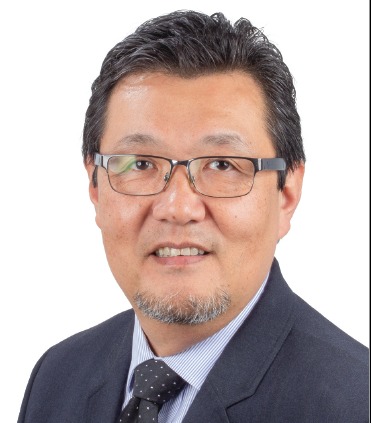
Those who do not know Lincoln Issamu Nojima may have the impression that he is a sedate professor of Orthodontics. However, this great professional has struggled and endured a difficult journey to become the orthodontist that he is today. In the beginning of his career, as he had no dependable advisor, he attended several short-duration courses. He took his first course on typodont in 1989. However, unsatisfied with his learning up to that time, he sought to improve his education by taking other courses to achieve the goal of being a good orthodontist. He even took a course at the Tweed Foundation in 1994. Until one day, his friend Geraldo Queiroz suggested that he should take the Orthodontics course at the Federal University of Rio de Janeiro (UFRJ), which he did. He went on to take a Master’s Degree (1995-1996) and a Doctorate (1999-2004) and started working as a professor in the Department of Orthodontics of UFRJ in 1997. In his search for more knowledge, he had the opportunity to take a Post Doctorate (2011-2012) in the Case Western Reserve University, Cleveland, OH, where he is currently a Visiting Associate Professor at the same Department of Orthodontics. He works in a research project in partnership with the Department of Orthodontics of the Yonsei University, Seoul, South Korea, with which he has a cooperation agreement. His line of clinical research investigates current topics, such as miniscrew-assisted rapid palatal expansion (MARPE), mini-implants, cone-beam computed tomography, orthodontic aligners and indirect orthodontic bracket bonding. However, during this promising, successful journey, he was not alone: he has had the unconditional support of his wife, Matilde, with whom he raised a beautiful family, with his sons Vitor Kenji and Pedro Eiji. A devoted father and husband, he loves to practice Kendo after a busy working day. He also enjoys fishing and walking with his family on weekends. It is an honor to have been invited to coordinate his interview, particularly because of the chance to talk about a serious, devoted, intelligent professional that is extremely proactive and an example to be followed.
